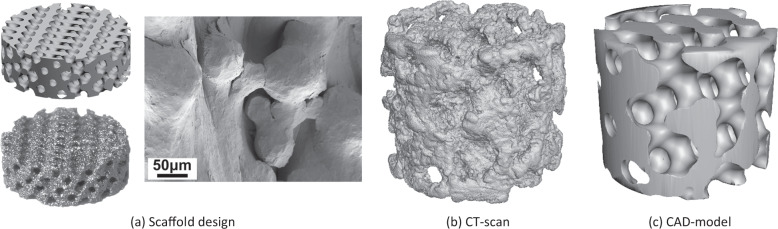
a Ti64-scaffolds are designed with a pore- and lattice- diameter of 800 µm. Representative images of CAD-model (top, left), EBM manufactured scaffold (bottom, left) and SEM image (right) of a large scaffold. b CT-scan of a manufactured small scaffold based of the CAD-model in c
